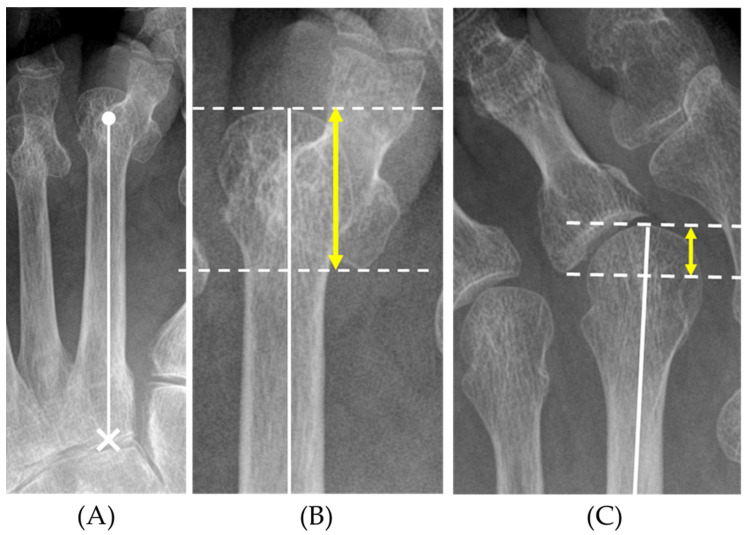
Definition of the MOD on plain radiographs. The metatarsal axis was defined as the line through the center of the metatarsal head (●) and the center of the proximal joint surface (×) (A). The MOD (↔) was defined as the distance between the tip of the metatarsal head and the most proximal point of the base of the proximal phalanx along the metatarsal axis (B). The MOD also reflects varus or valgus deformity to some extent (C). MOD: metatarsophalangeal overlap distance.
